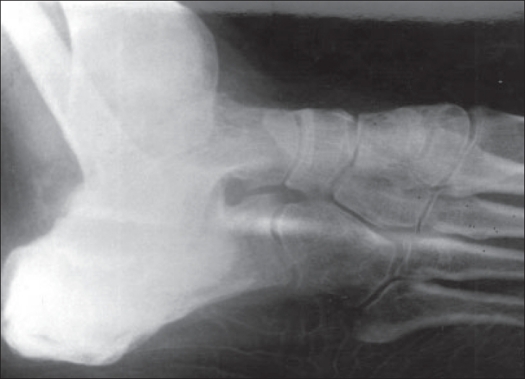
Postoperative X-ray after extended curettage and filling of the cavity with bone cement
